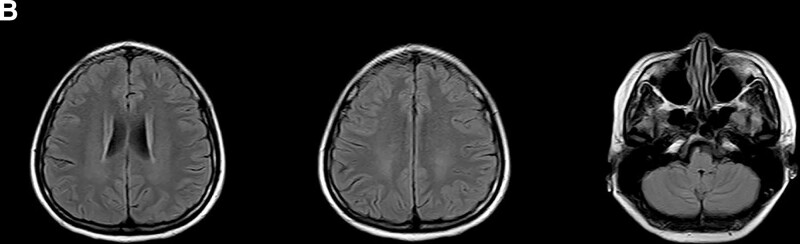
The follow-up MRI showed a decrease of the white matter lesion after 3 months and complete recovery at postoperative 6 months (B). MRI = magnetic resonance imaging.
